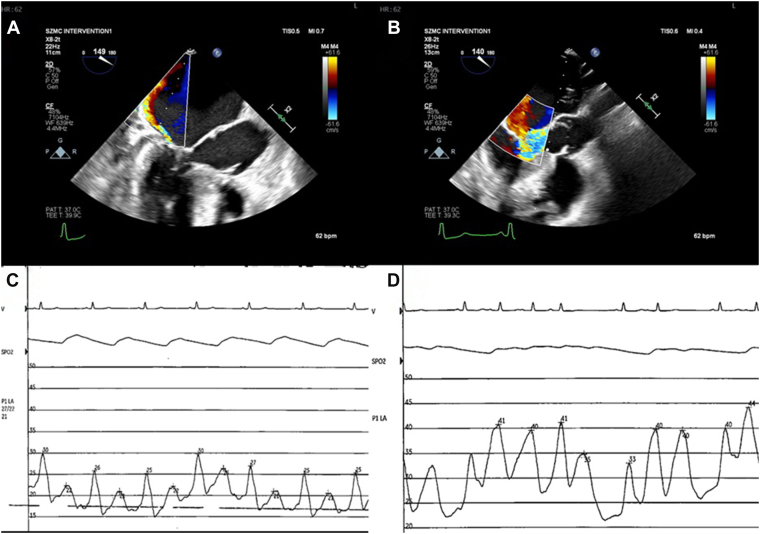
Hemodynamics in systolic anterior motion mitral regurgitation. Transesophageal echocardiographic images and left atrial pressure measurements at the beginning of the procedure: (A, C) during high blood pressure vs (B, D) low blood pressure. A drop in blood pressure is associated with an increase in left atrial pressures and worsening mitral regurgitation.
